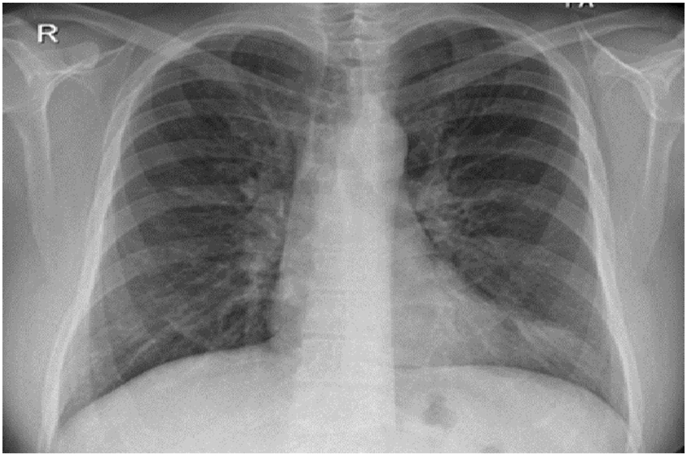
Chest x-ray PA, February 2018: Bilateral, perihilar, peribronchial cuffing with diffuse bilateral alveolar shadows more in the right lung.
